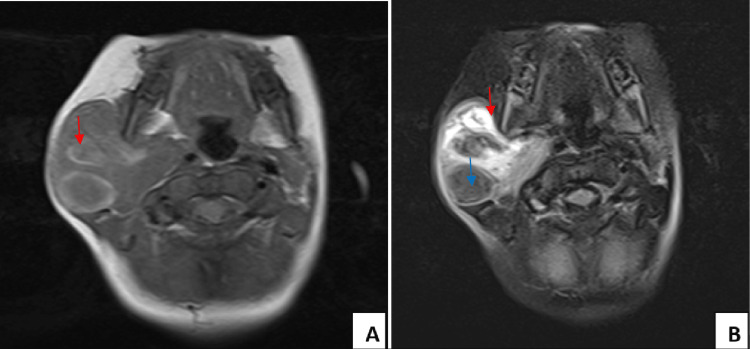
Facial MRI A: T1 sequence: Right latero-cervical lesional process centered on the right parotid region, fairly well limited, with a heterogenous hyposignal intensity on T1 weighted image. B: T1 sequence after injection of gadolinium: intensely and heterogeneously enhanced after injection of godalinium, delimiting large areas of necrosis (hyposignal T1; blue arrow) and stigmata of hemorrhage (hypersignal T1; red arrow).
